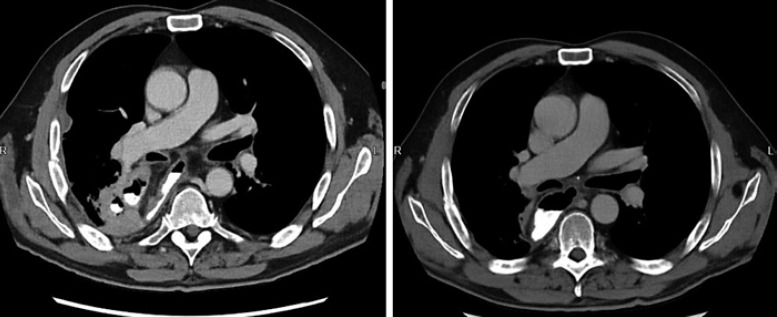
Comparable views of CT scan before (left) and after (right) the EPF closure.
